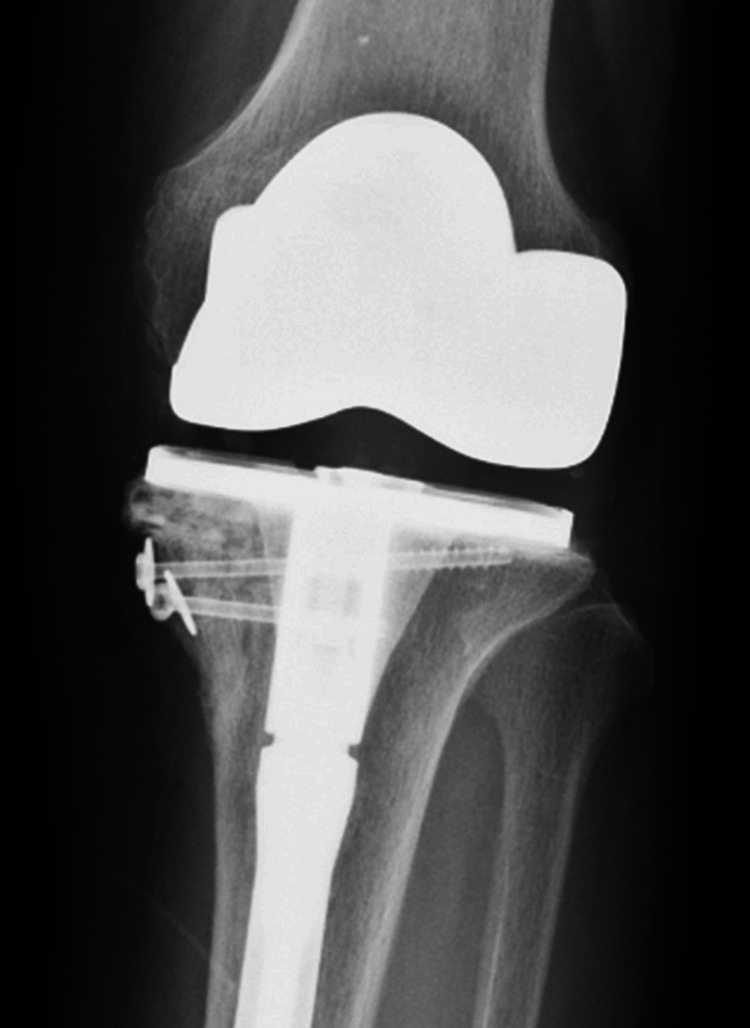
Two years postoperative radiography of TKA (AP view) TKA: total knee arthroplasty, AP: anteroposterior.
